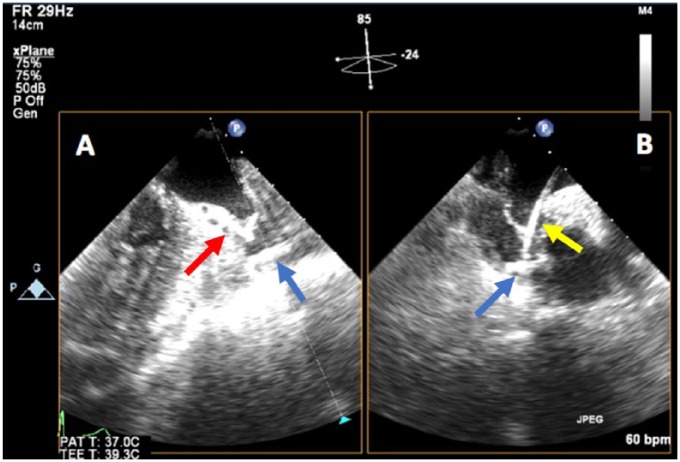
Transesophageal echocardiography images showing the Watchman device located in the patient’s transverse sinus. (A) Crown of the Watchman device (blue arrow) can be seen in the transverse sinus beyond the wall of the left atrial appendage (LAA; red arrow). (B) The sheath (yellow arrow) was not in the plane of view in image “A” but can be seen passing through the LAA into the transverse sinus in image “B.” The Watchman device (blue arrow) remains connected to the delivery system. Transverse sinus is showing evidence of fluid accumulation relative to preprocedure images (not shown).
